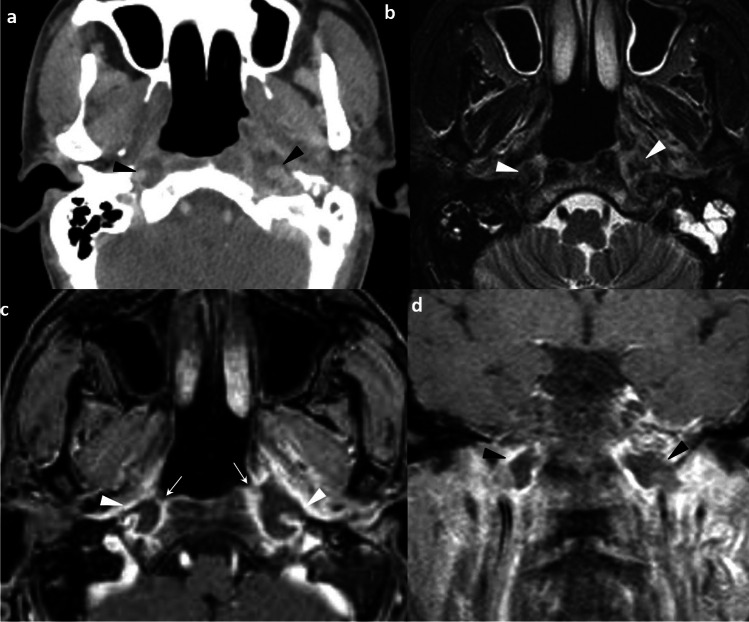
Post-radiation nasopharyngeal necrosis in a 49-year-old man with NPC (clinical stage T4N2M0). He developed epistaxis and progressive headache 6 months after radiotherapy. Nasoendoscopy showed crusted and ulcerated mucosa without mass. Axial contrast-enhanced CT image shows mucosal irregularity in bilateral nasopharynx. Rim-enhancing lesions are seen in bilateral nasopharynx with extension to carotid spaces encasing the internal carotid arteries (arrowheads) (a). Axial T2-weighted image with fat suppression shows the lesions to be heterogeneous in signal intensity (arrowheads) (b). Axial and coronal post-contrast T1-weighted image with fat suppression show focal defects in the bilateral nasopharynx as discontinuity of the deep mucosal line (arrows). The rim-enhancing lesions encasing the bilateral internal carotid arteries are again seen (arrowheads) (c) and (d). Deep biopsy was suspended due to the close relationship with internal carotid arteries. The lesions gradually resolved on conservative management with restoration of the continuity of the deep mucosal white line on follow-up MRI (not shown)
